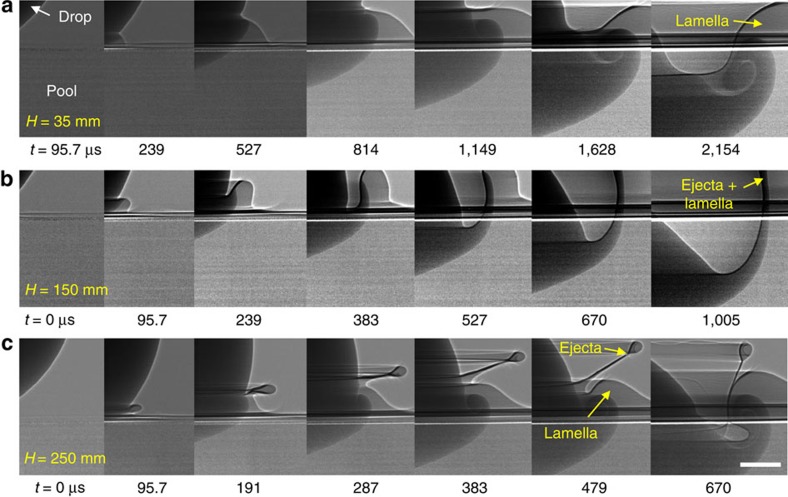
X-ray images of jetting phenomenon in drop impact. Here water–ethanol (4:6 in mass fraction) drops are used. (a) Impact at *H*=35 mm (*U*=0.83 m s^−1^) with We=50 and Re=724. Only a lamella is formed, and the vortex ring is generated within the lamella. (b) Impact at *H*=150 mm (*U*=1.71 m s^−1^) with We=214 and Re=1,498. An ejecta is merged with the lamella, suppressing the formation of a vortex ring. (c) Impact at *H*=250 mm (*U*=2.21 m s^−1^) with We=356 and Re=1,934. The drop diameter is 2.3 mm. An ejecta and a lamella separately emerge, and the vortex ring is formed within the lamella. Scale bar, 300 μm long.
